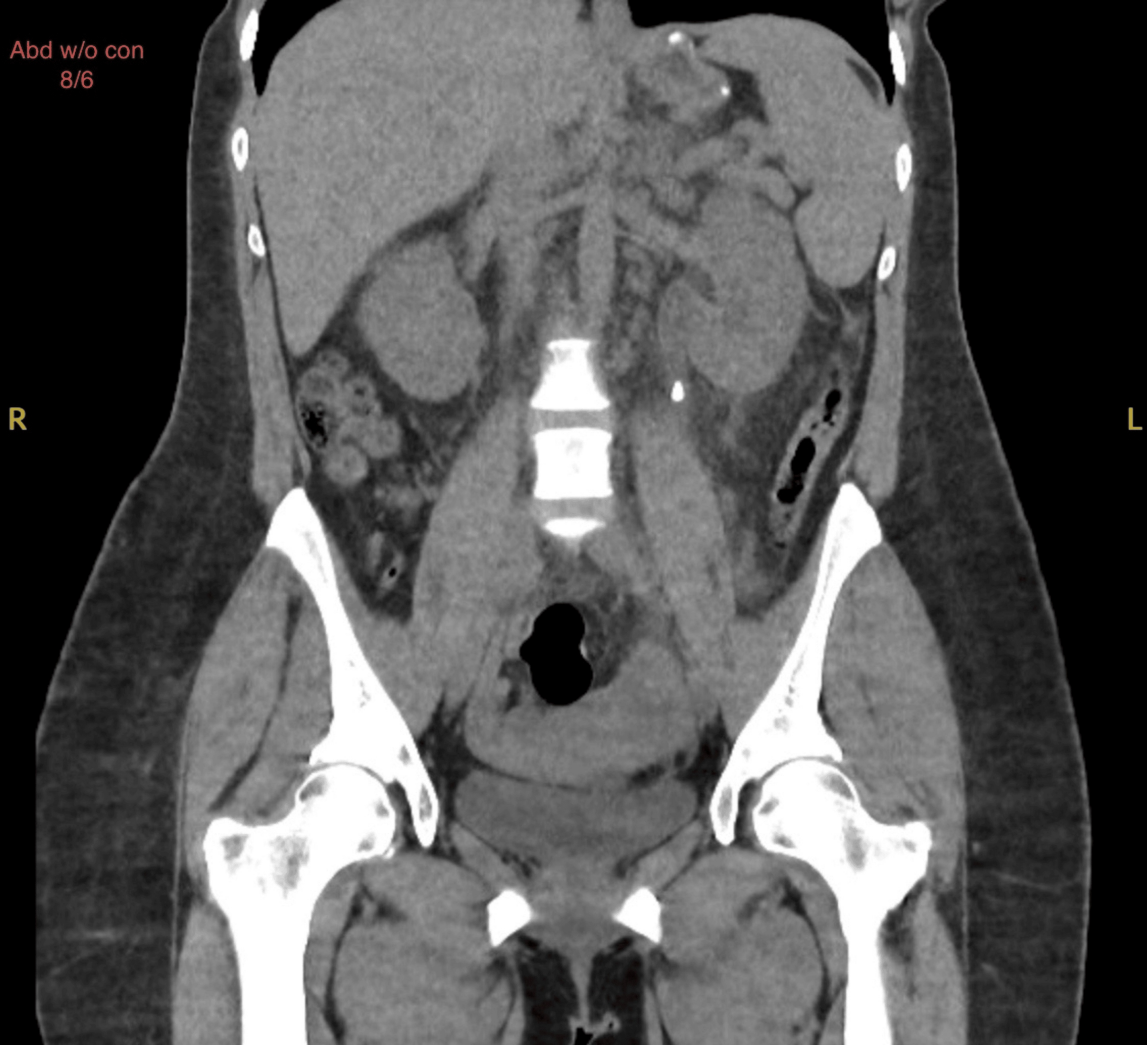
Coronal CT abdomen and pelvis without contrast showing moderate left hydroureteronephrosis (red arrow) and trace left perinephric fluid (yellow arrow) indicative of calyceal rupture The CT scan is a coronal view of the abdomen without contrast (Abd w/o con), dated 8/6. "R" indicates the patient's right side, and "L" indicates the patient's left side.
